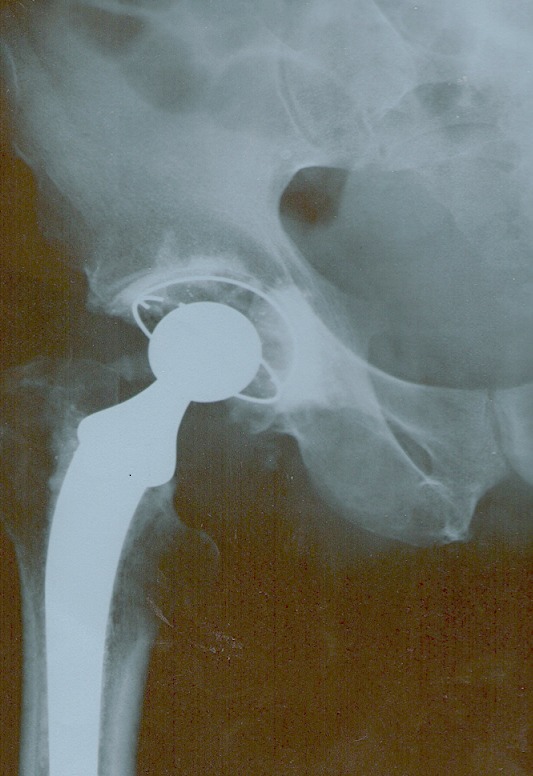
Radiographie post-opératoire de la hanche du 2ème patient
